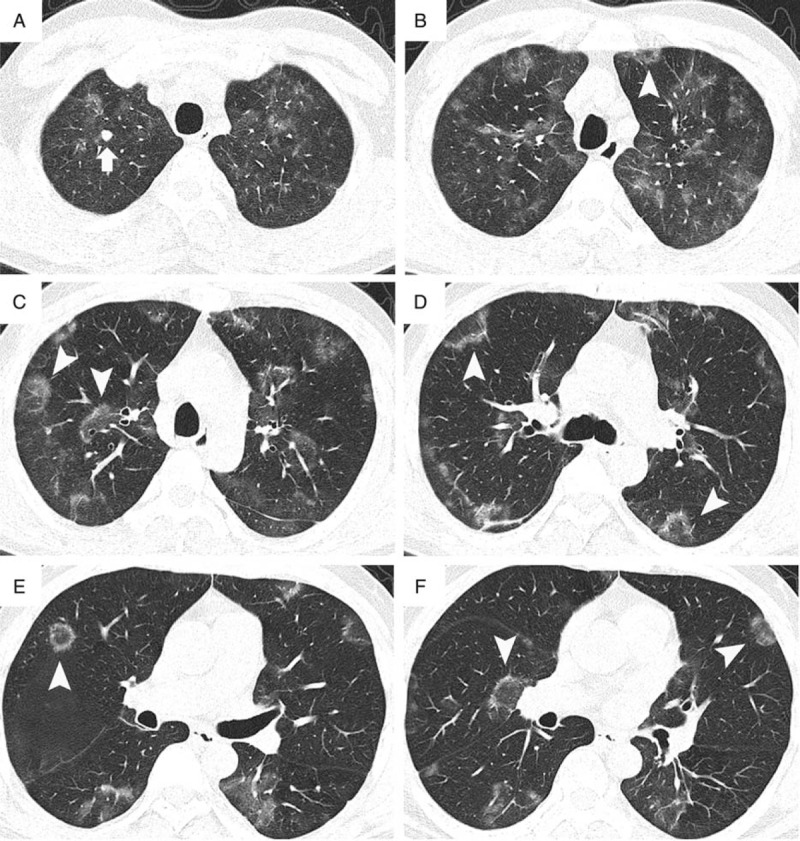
Imaging of the lung. (A) High-resolution computed tomography scans demonstrate multiple ground-glass opacities bilaterally in the uppers lung and a small nodule (arrow) in the right upper lung. (B–F) High-resolution computed tomography scans demonstrate bilateral patchy ground-glass opacities, multiple lesions of the reversed halo sign (arrowhead).
